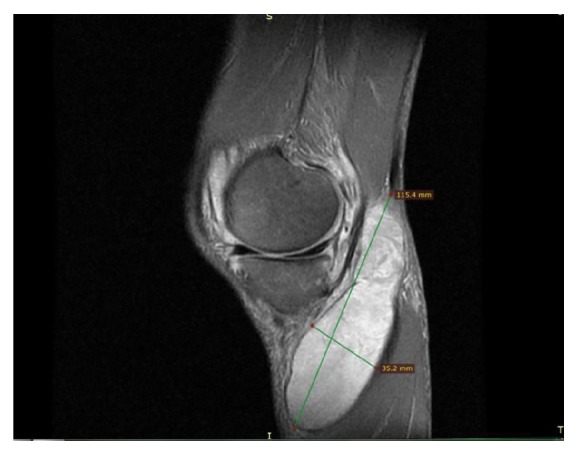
Magnetic resonance imaging sagittal plane view: cyst size.
